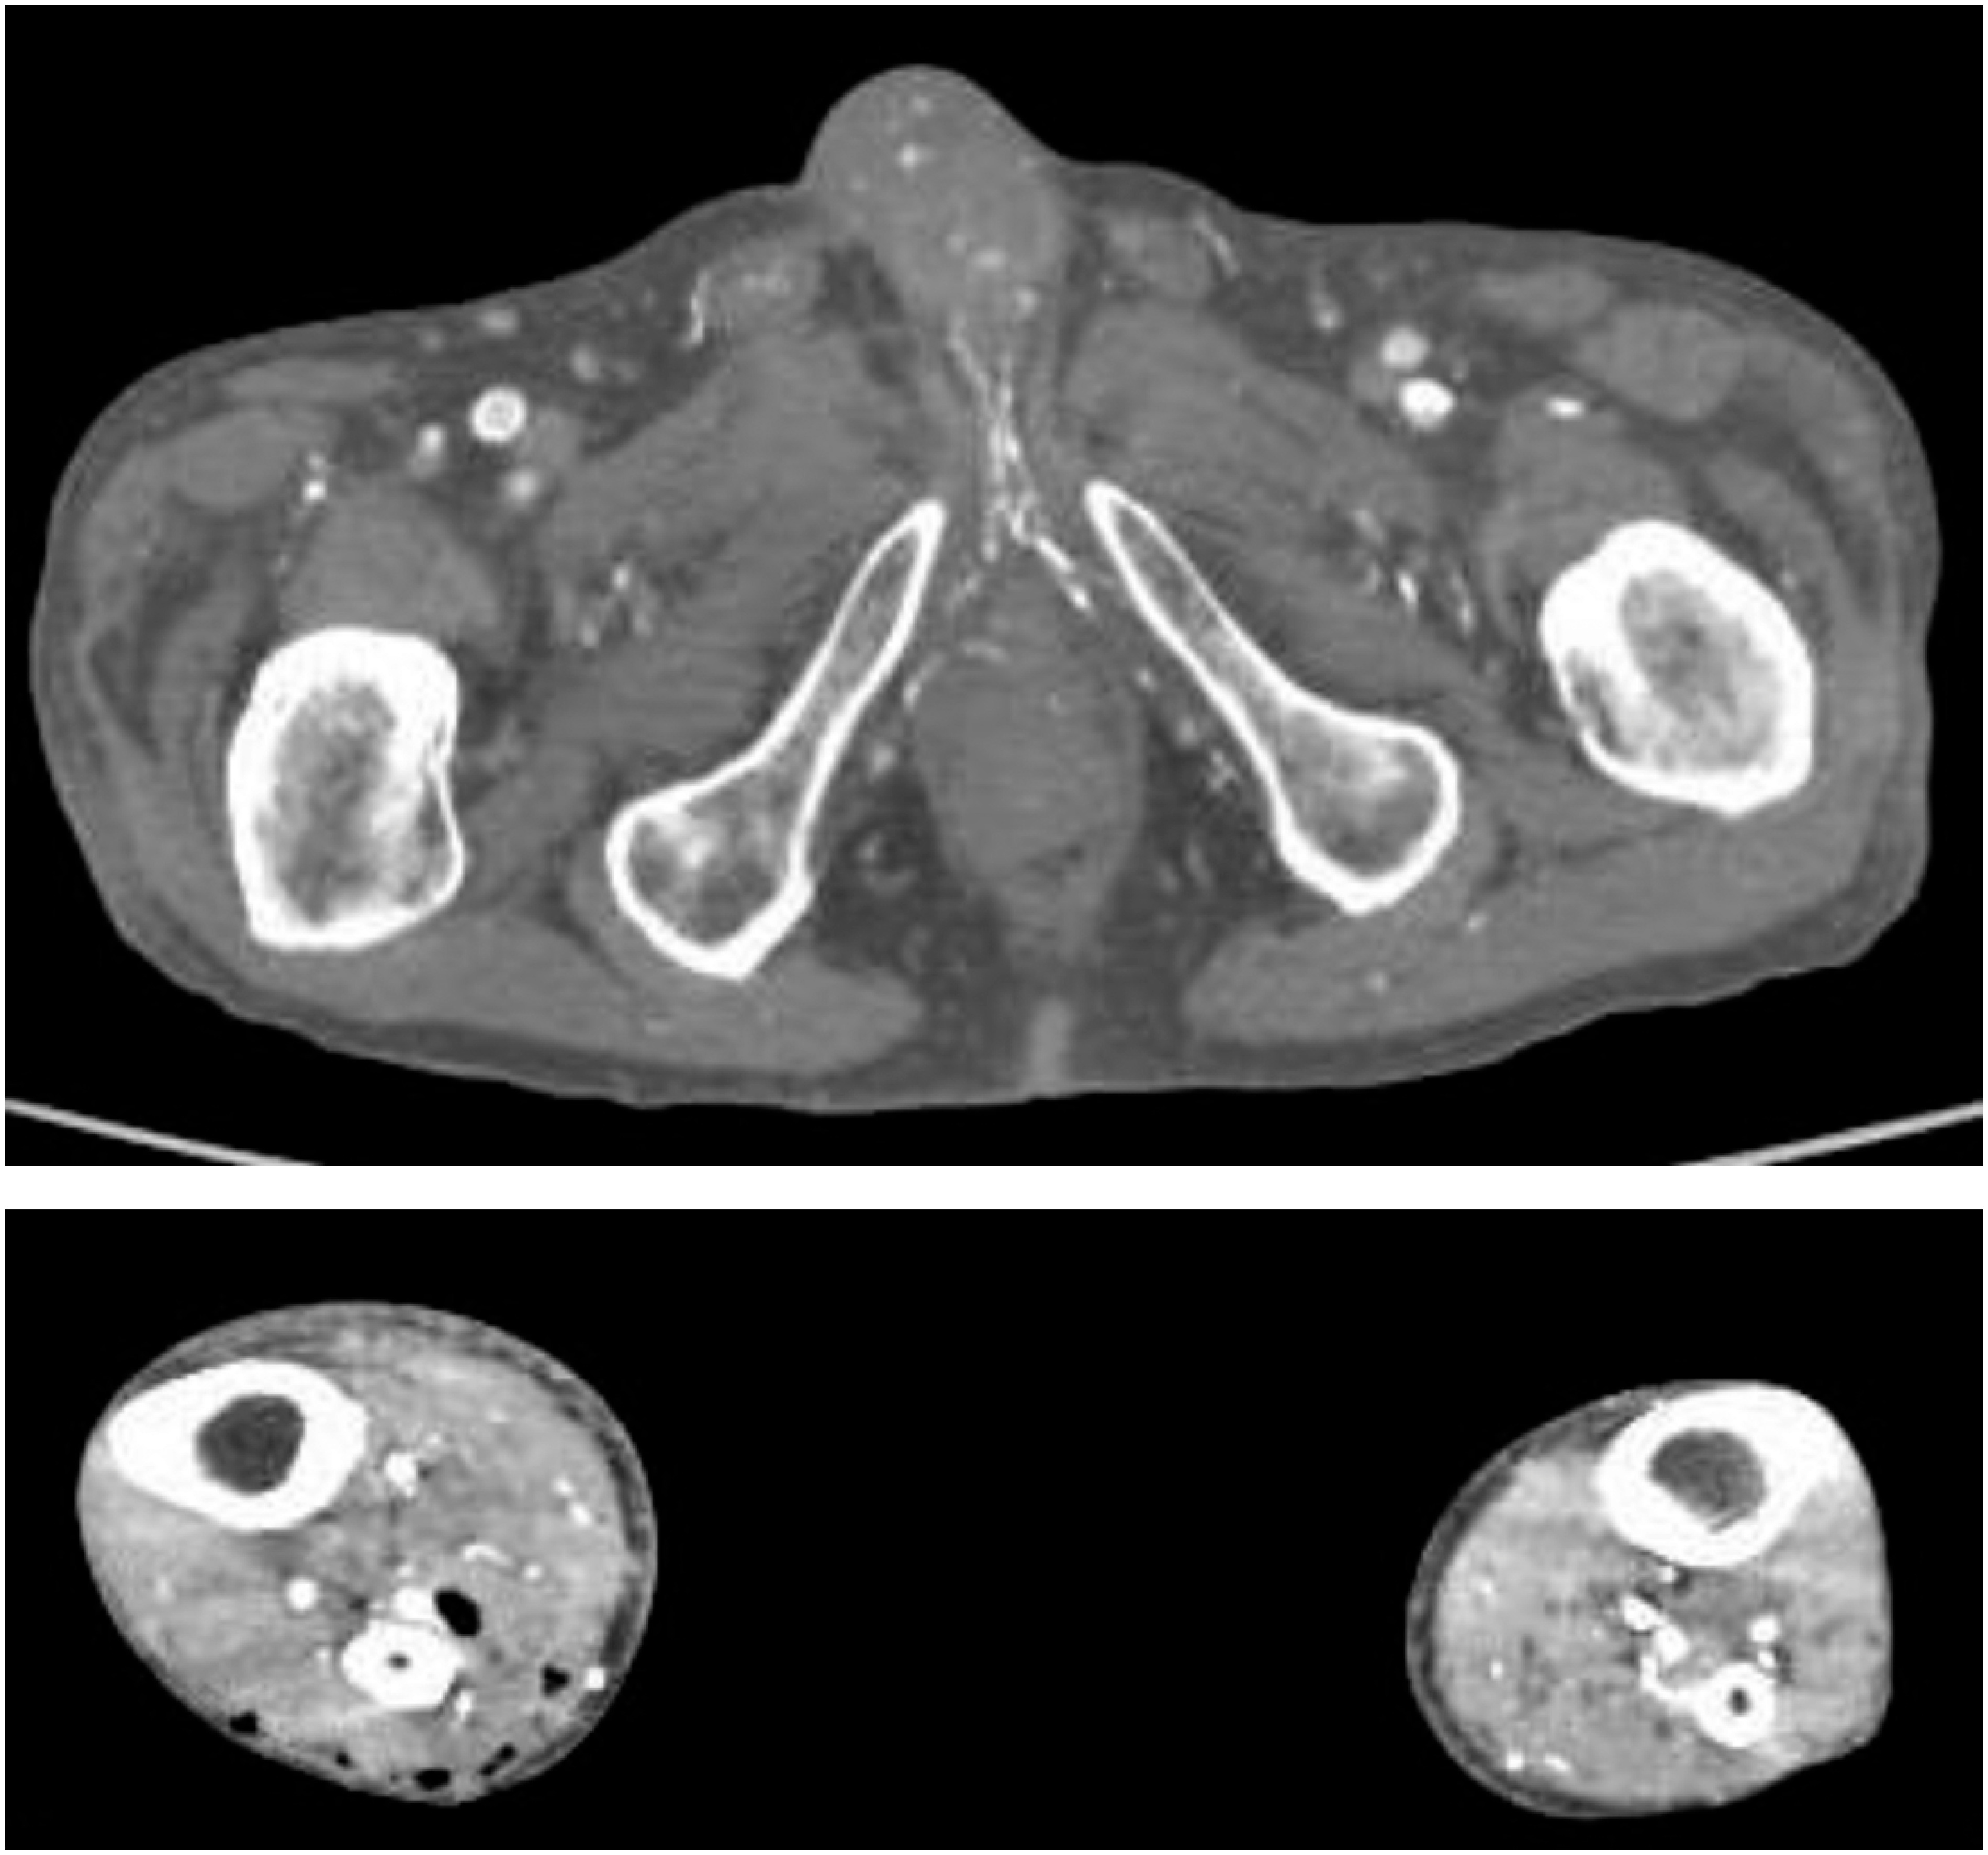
Fig. 2 Computed tomography showing penile and femoral artery calcifications. Gaseous cellulitis is noted in the right lower leg.
